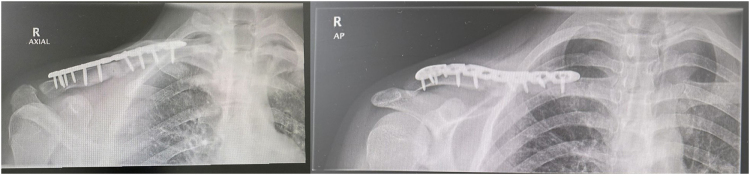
Postoperative right clavicle X-rays (AP and axial views), 31 July 2025, showing united fracture with well-aligned plate and screws.
